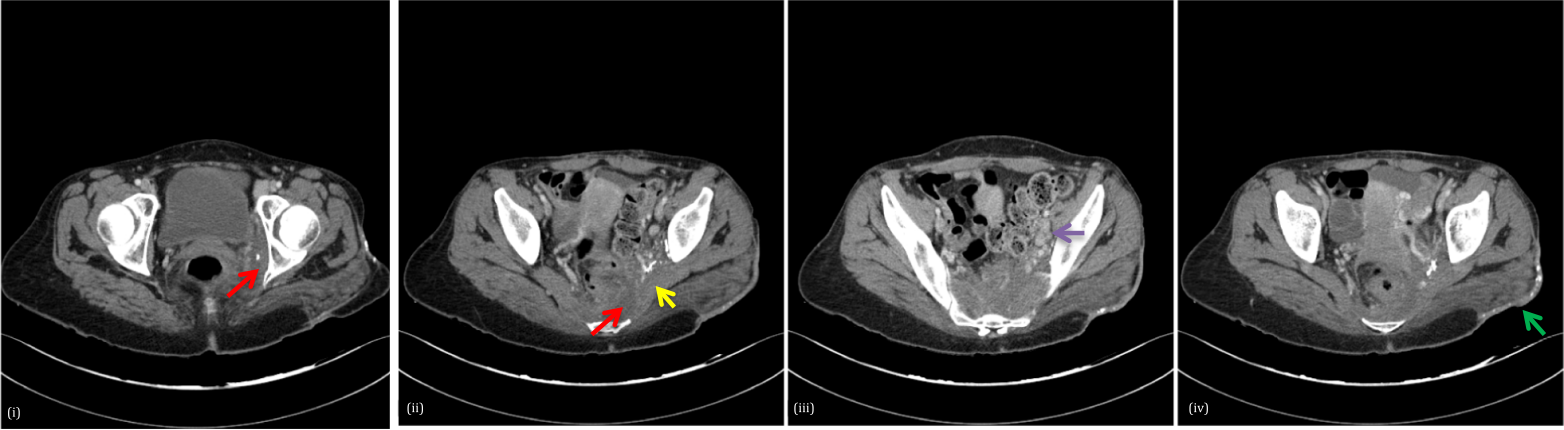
Computed tomography of the thorax, abdomen, and pelvis with contrast performed when the patient presented with foot drop. Serial axial computed tomography images: (i) and (ii) demonstrate an infiltrative heterogeneous soft tissue mass-like abnormality involving the left pelvic sidewall and presacral space (red arrows) intimately involving the left obturator internus and piriformis muscle (yellow arrow). Dystrophic areas of calcification are seen within the soft tissue mass. Despite the ill-defined and infiltrative nature, there is no associated bony destruction. Image (iii) depicts left iliac chain lymphadenopathy (purple arrow); image (iv) shows a loss of the normal subcutaneous fat, soft tissue thickening and multiple, punctate calcifications overlying the left gluteal maximus muscle, suggesting a chronic process (green arrow)
